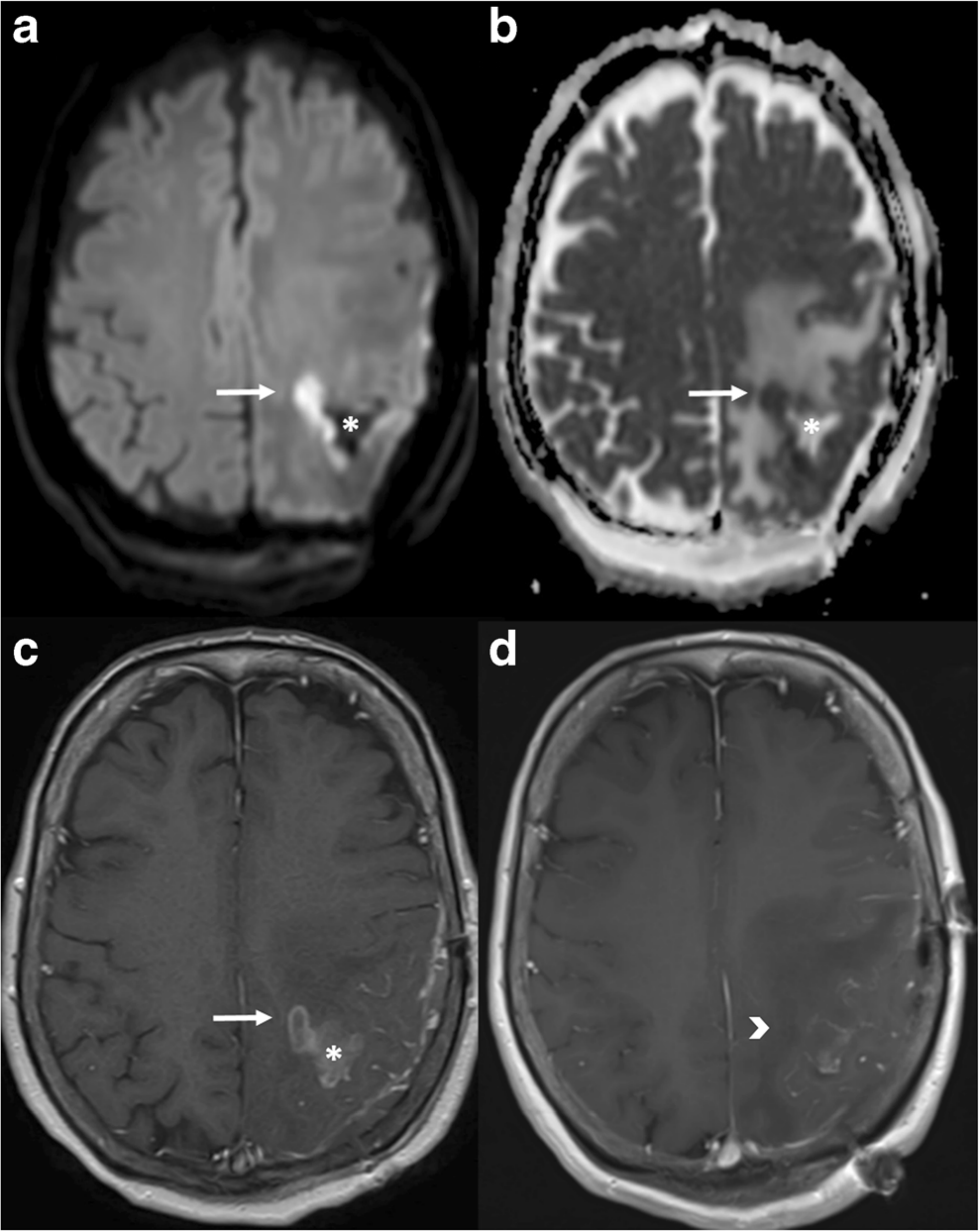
Post-surgical ischaemia. Immediate follow-up MRI in a patient who underwent surgery for resection of a suspicious enhancing mass. In the medial aspect of the resection cavity (asterisk) there is an enhancing area on the T1 post-contrast sequence (c, arrow). This finding alone could represent residual tumour, but the presence of restricted diffusion with high signal on DWI (a) and a low ADC value (b) meant that a small area of peri-surgical ischaemia was more likely. Three-month follow-up T1 post-gadolinium MRI (d) shows absence of enhancement in the same region (arrowhead), confirming this diagnosis
